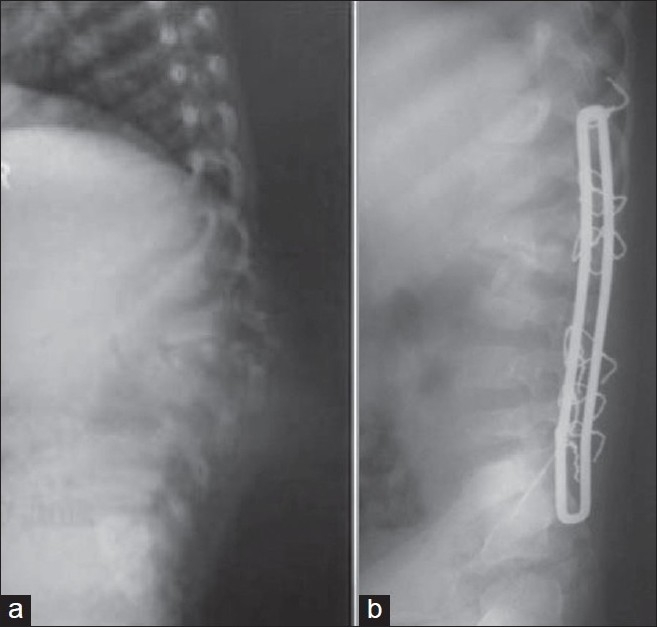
Lateral X-ray of lumbosacral spine (a) in a four-year-old child shows TB of L1–L3 with kyphosis of 40°. Lateral X-ray of same patient (b) where lumbar kyphosis is corrected with pedicle subtraction and Hartshill fixation. The kyphosis is corrected to 10°
